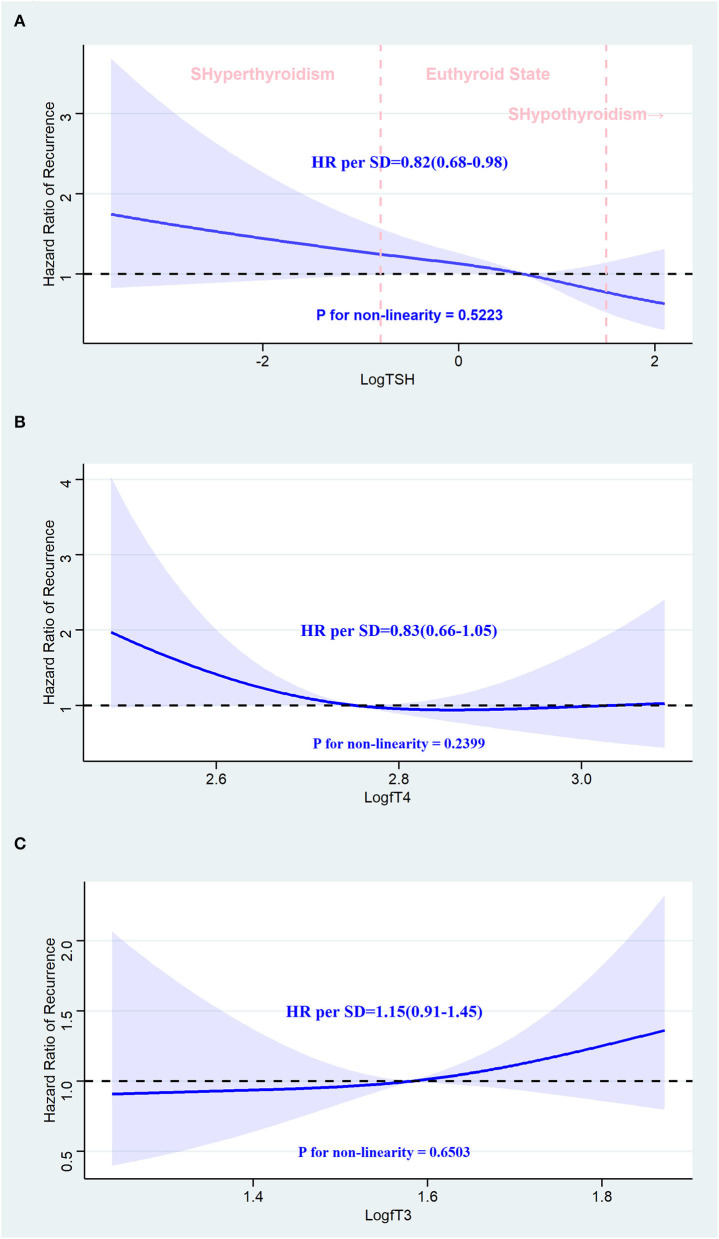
Association between thyroid hormone and recurrence of atrial fibrillation after radiofrequency catheter ablation using a restricted cubic spline regression model. (A) TSH and recurrence of AF after RFCA. (B) fT4 and recurrence of AF after RFCA. (C) fT3 and recurrence of AF after RFCA. Results are adjusted for age, BMI, gender, current smoking and drinking status, AF pattern, CHA2DS2-VASc score, AF duration, echocardiogram information, past diagnoses, current medications laboratory tests, and procedure parameters. The restricted cubic spline regression model is conducted with three knots at the 10th, 50th, and 90th percentiles of TSH, fT4, and fT3, and they are modeled with Log transformation. The blue ribbon represents the 95% CI for the spline model. Reference lines for no association are indicated by the dashed black lines at an HR of 1.0. HR, hazard ratio; CI, confidence interval; SD, standard deviation; SHyperthyroidism, subclinical hyperthyroidism; SHypothyroidism, subclinical hypothyroidism; AF, atrial fibrillation; RFCA, radiofrequency catheter ablation.
